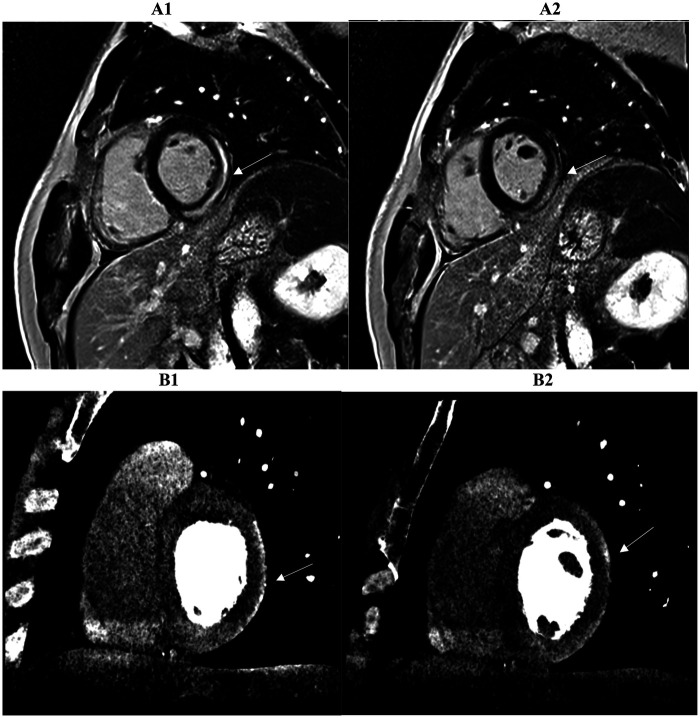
Two consecutive basal and mid-basal short-axis images demonstrate a typical subepicardial scar of the inferolateral wall highlighted by late iodine enhancement (LIE) (B1, B2). The CCTA findings suggested myocarditis and were confirmed by cardiac MRI performed within 14 days of the acute event. A subepicardial-intramural stria of late gadolinium enhancement (LGE) is seen on the basal and mid-basal short-axis LGE images (A1, A2), with 83% spatial agreement between the two techniques based on the matching presence or absence of myocardial enhancement in the corresponding segments (arrow).
